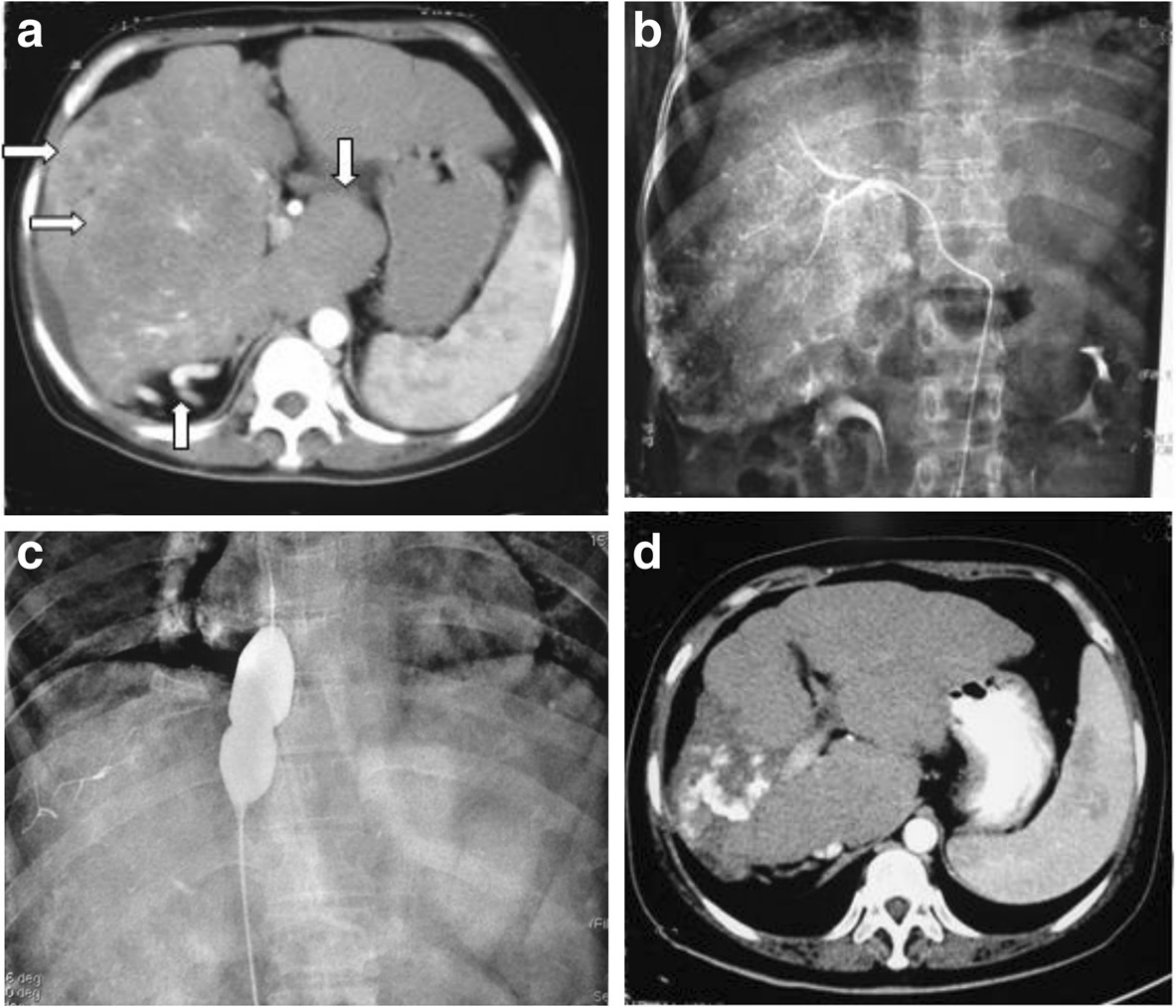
45-year-old woman with HCC in BCS. (a) Contrast-enhanced transverse CT scan showing two large heterogeneous enhanced nodules (⇨) in the right liver during arterial phase and enlarged caudate lobe (⇩), ventral varicosities (⇧), liver cirrhosis and mild ascites. (b) The two nodules were given TACE. (c) The blocked inferior vena cava was given angioplasty. (d) CT scan showing that the nodules were obviously shrinked and obtained stable effect after 4 TACE and 1 angioplasty during the 22-month treatment. Lipiodol was not located equably in the nodules.
